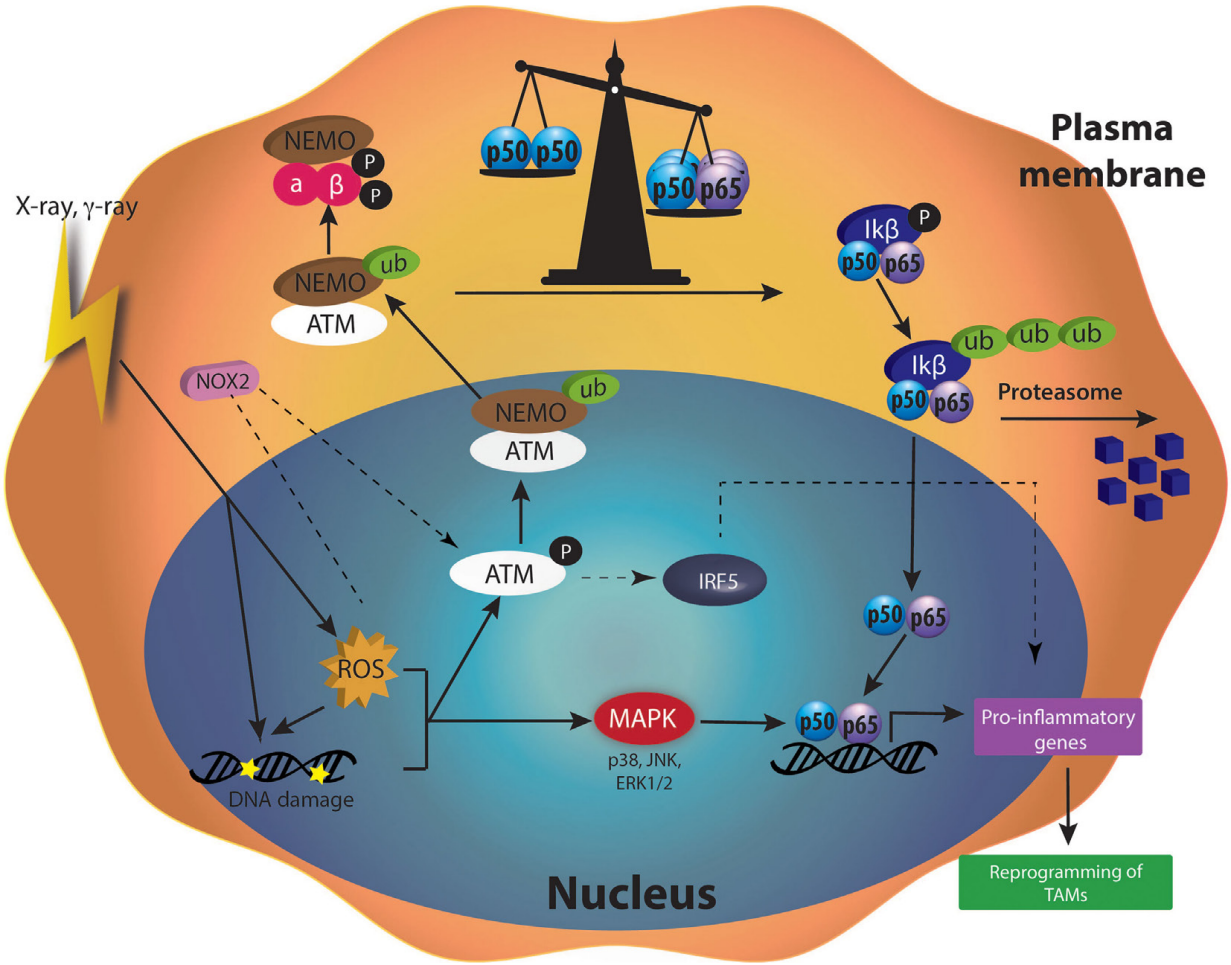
Molecular mechanisms activated after moderated doses of irradiation in tumor-associated macrophages (TAMs). Ionizing radiation (X-rays or γ-rays) induces DNA damage and elevated reactive oxygen species (ROS) content in cells. DNA repair machinery [such as ataxia telangiectasia mutated (ATM)] is activated by DNA damage and initiates the ubiquitination of NFκB essential modulator (NEMO), a subunit of the IKK complex. Therefore, ubiquinated NEMO can drive the activation of IKK complex in the cytoplasm. The degradation of IκB protein by the proteasome allows the release of p50–p65 nuclear factor kappa B (NFκB) in the cytoplasm. p50–p65 NFκB is then translocated into the nucleus and induces the transcription of pro-inflammatory genes, leading to the reprogramming of TAMs. ROS are also able to stimulate mitogen-activated protein kinase (MAPKs). Once phosphorylated, MAPKs also participate to the activation of NFκB and hence, to the transcription of pro-inflammatory genes.
